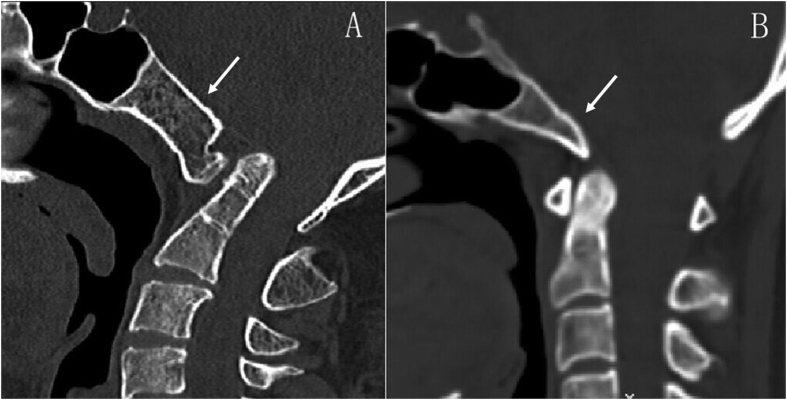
The clivus (white arrows) shown on the sagittal CT images in patients with (A) and without (B) C1 assimilation.
